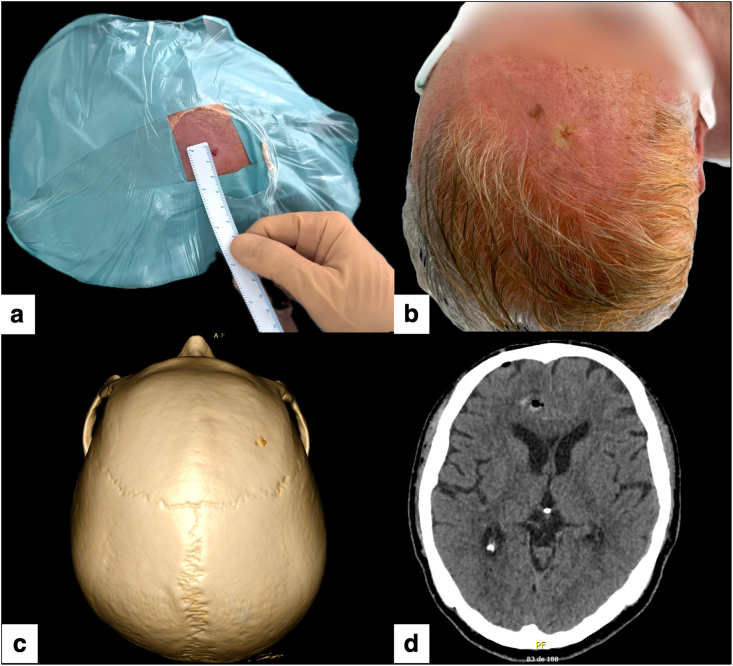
Postoperative wound appearance, micro-burr hole visualization, and radiological control. (a) Immediate postoperative view showing a single pinpoint entry site of only a few millimeters, without a conventional scalp incision. (b) Final aspect of the minimal-access robot-assisted biopsy, showing a single fine suture and no visible linear incision. (c) 3D CT reconstruction revealing the 2.7 mm micro-burr hole on the right frontal bone, barely visible on the skull surface. (d) Axial postoperative CT scan confirming correct biopsy trajectory and absence of significant hemorrhage or mass effect.
